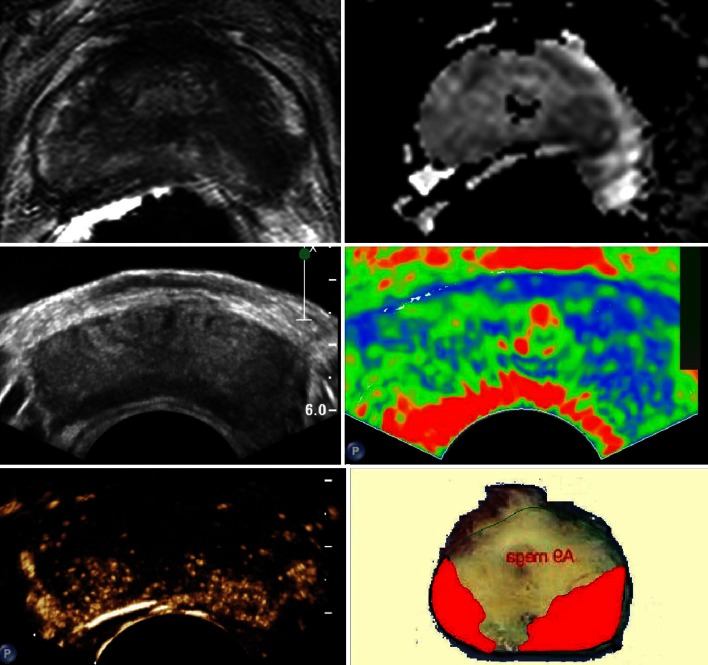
Multiparametric ultrasound and MRI modalities. *Top left *and *right* T2-MRI and diffusion-weighted MRI indicating tumour presence on the *left* side. *Middle left *and *right* GSU and elastography indicating tumour presence on the *left* side. *Bottom left *and *right* DCE-US and pathology indicating tumour presence on both sides
